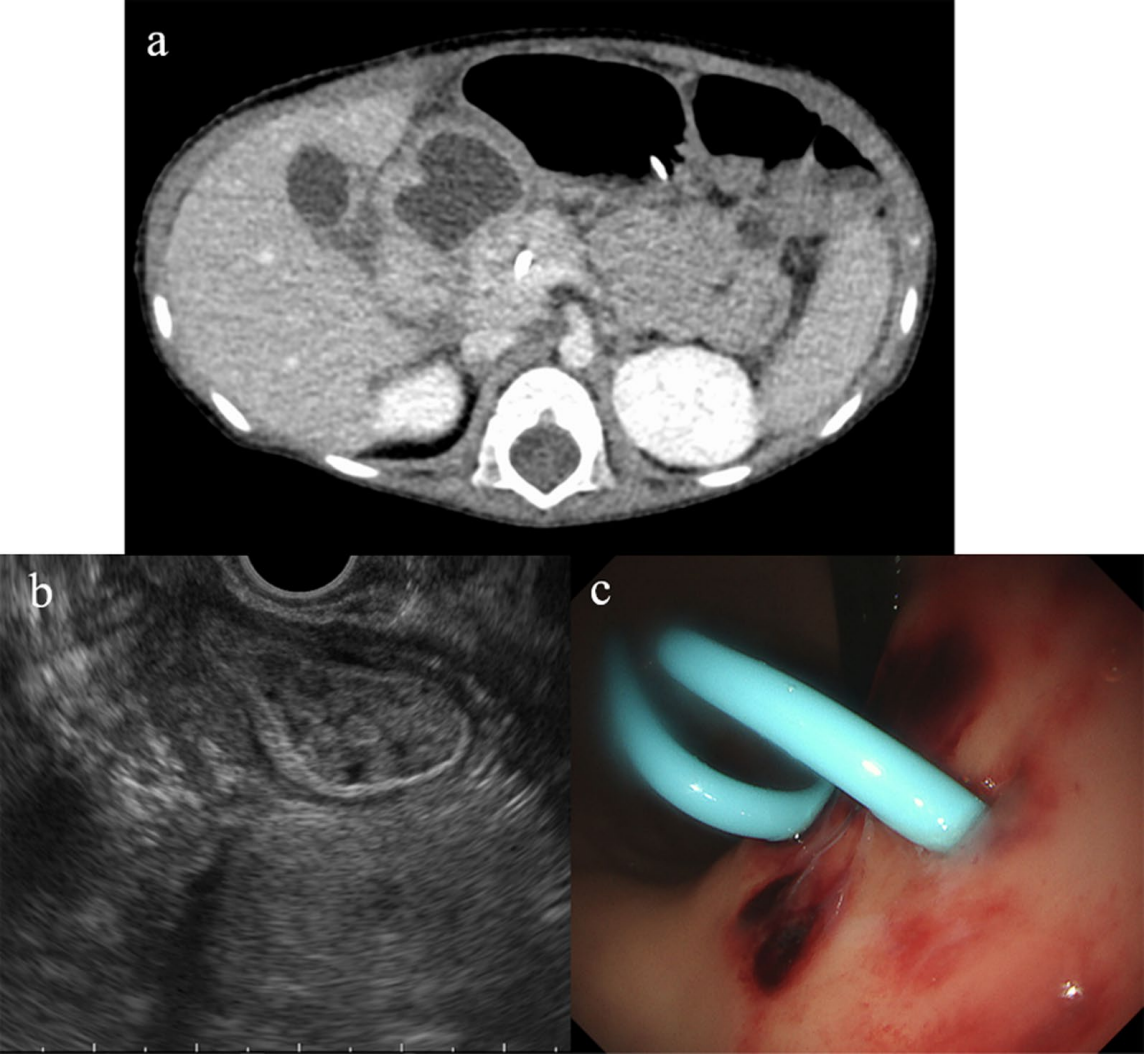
Endoscopic transgastric drainage of the pancreatic pseudocyst. Enhanced computed tomography before endoscopic drainage depicted the pseudocyst directly adjacent to the stomach; it was 28 × 20 mm in size (a). It was punctured under endoscopic ultrasound guidance (b), and a 7-Fr pigtail catheter was placed in the gastric tract to serve as a stent (c)
